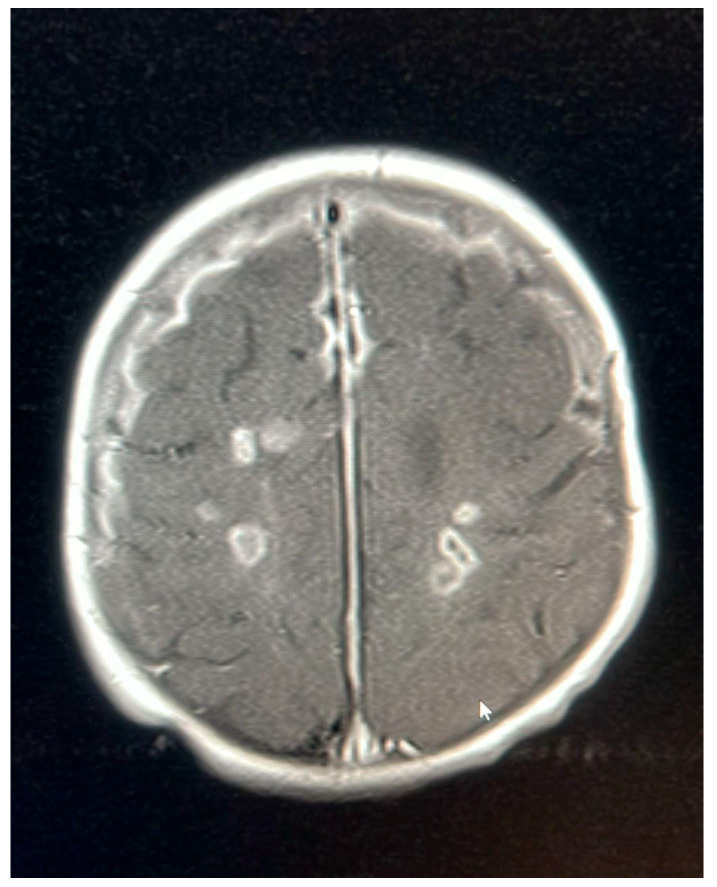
MRI brain scan showing periventricular lesions.
